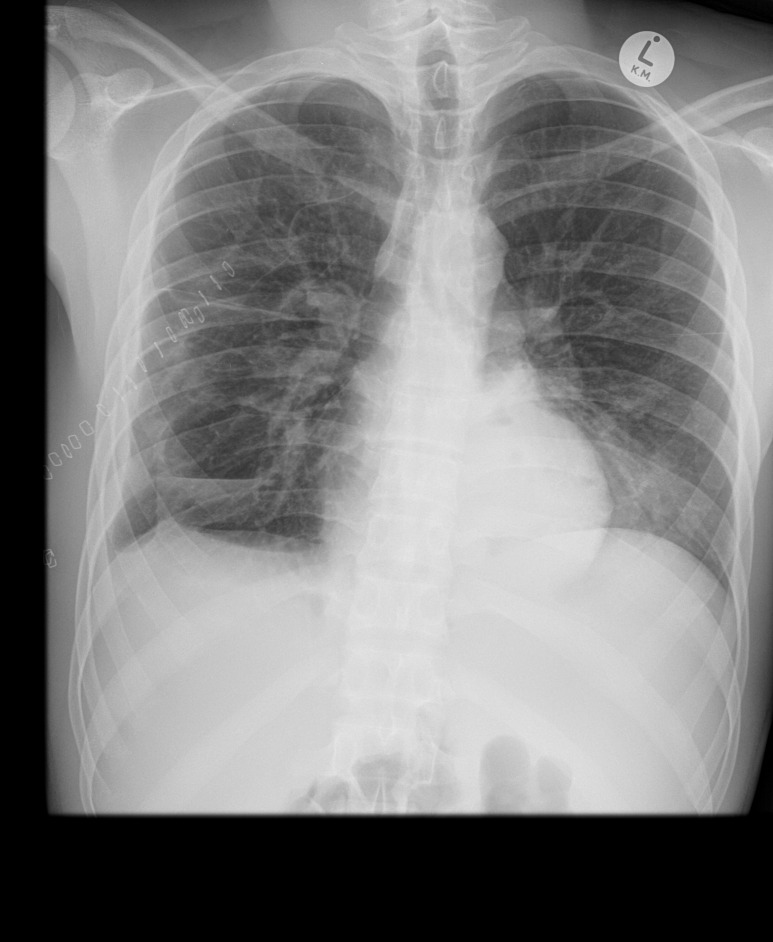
Chest radiograph on day 8 post right thoracotomy
